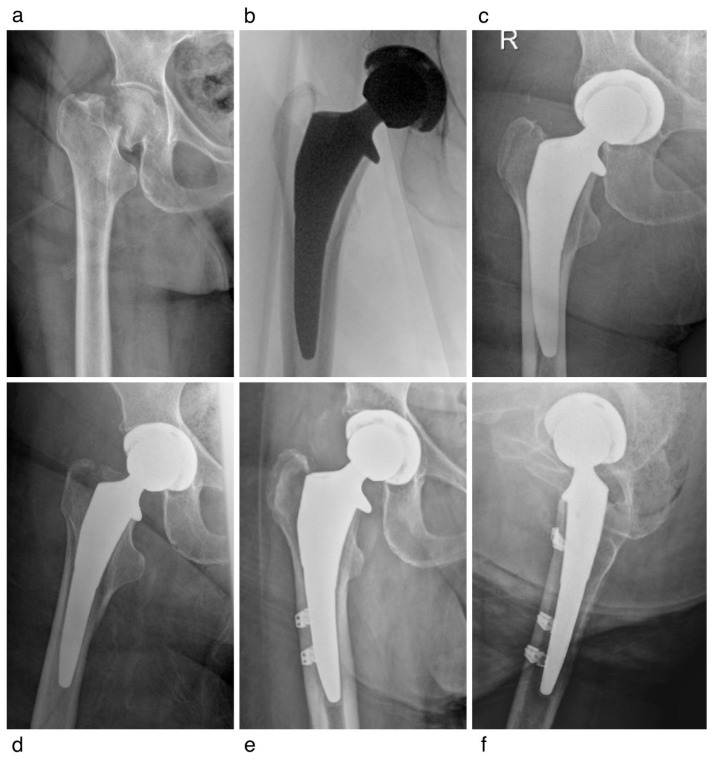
A 90-year-old woman initially presented with a femoral neck fracture. One week after primary fixation, a fall resulted in a periprosthetic Vancouver B1 fracture. The fracture was treated with three cerclage cables and demonstrated radiographic union at 3 months postoperatively. (a) Initial femoral neck fracture, anteroposterior (AP) view (b) Intraoperative AP view (c,d) Eight days postoperatively, showing Vancouver B1 periprosthetic fracture, AP and frog-leg lateral views (e,f) Three months postoperatively with healed fracture and three cerclage cables, AP and cross-table lateral view.
